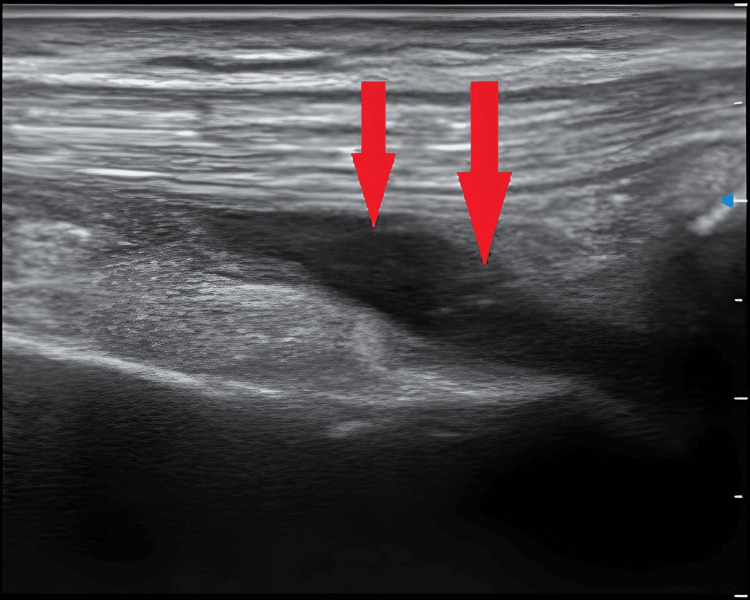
Ultrasound of the left knee demonstrating a collection in the quadricipital fossa
